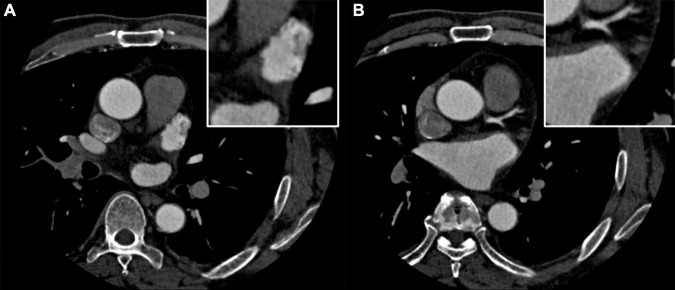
(A,B) Preoperative left atrium computed tomography (CT) angiography excludes the intracardiac thrombus.
